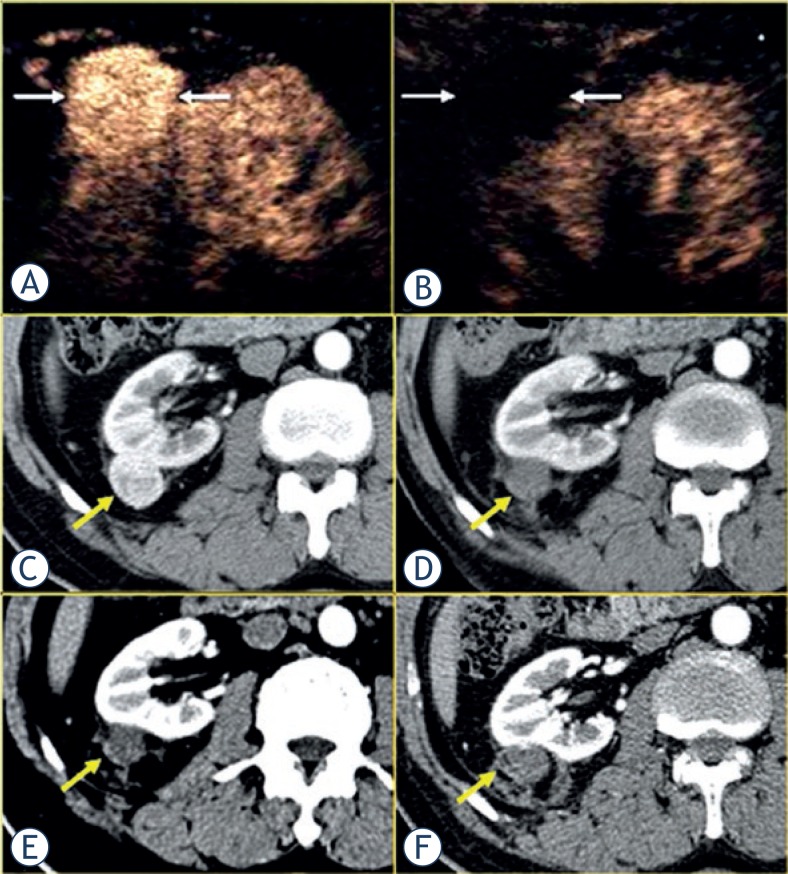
Images in 82-year-old female with a 3.9 cm × 3.7 cm renal clear cell carcinoma treated with microwave ablation (MWA). (A) Pre-ablation contrast-enhanced US scan showed one hyper-enhancement lesion exophyticly (arrow). (B) Seven days after ablation contrast-enhanced US showed the whole lesion no-enhancement continuously. (C) Transverse contrast-enhanced multidetector-row CT imaging showed one hyper-intense lesion pre-ablation and hypo-intense one in arterial phase 7 days (D), 1 month (E) and 6 months (F) after ablation.
